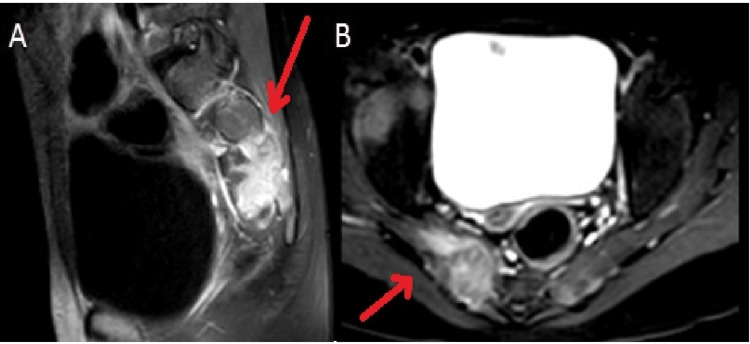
MRI of the lumbar spine showing an osseous metastatic deposit involving the right sacral ala with an associated enhancing extraosseous soft-tissue mass extending into the adjacent sacral neural foramena and causing nerve root compression. (A) Sagittal view. (B) Axial view.
